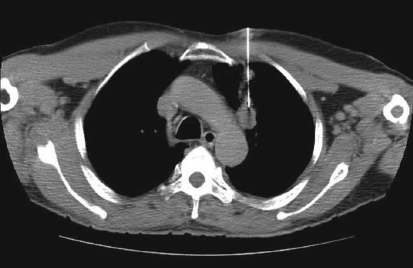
Transthoracic approach for biopsy of prevascular node. Biopsy results revealed metastases from adenocarcinoma
